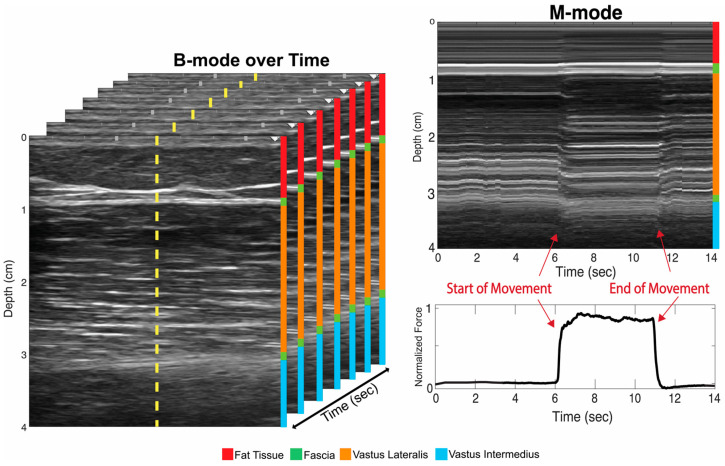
A set of clinical B-mode ultrasound images taken over time with a single scan line (yellow). On the left, an M-mode image created from the yellow scanline on the B-mode stack on the right over time. The start of the movement and end of movement is labeled (red arrows) as the M-mode in relation to the force trace.
